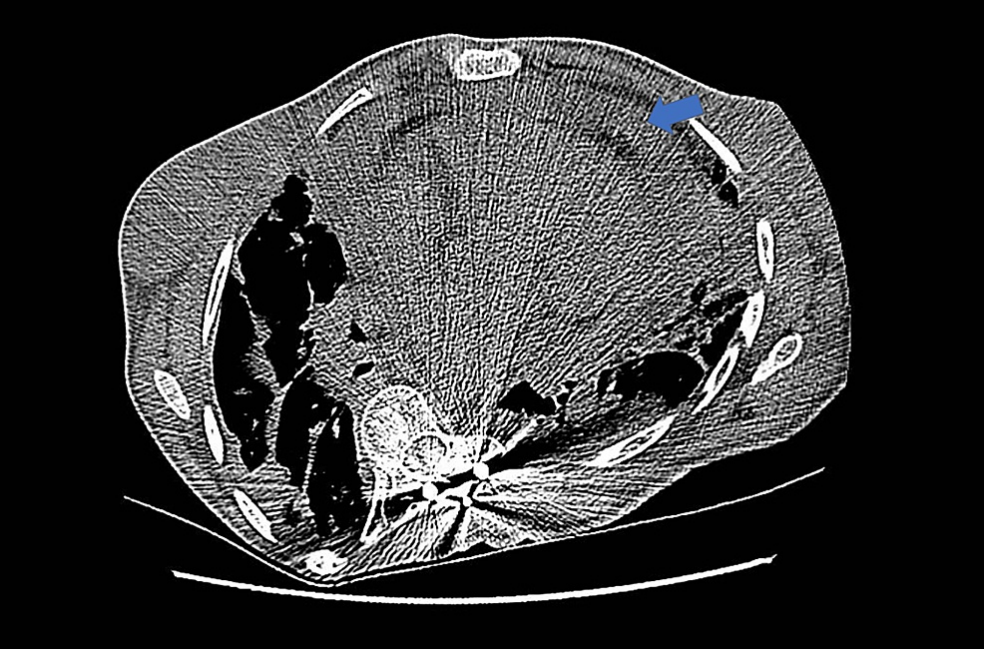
The chest CT shows bibasilar consolidative opacities indicating an infection. A new moderate pericardial effusion is seen (blue arrow).
